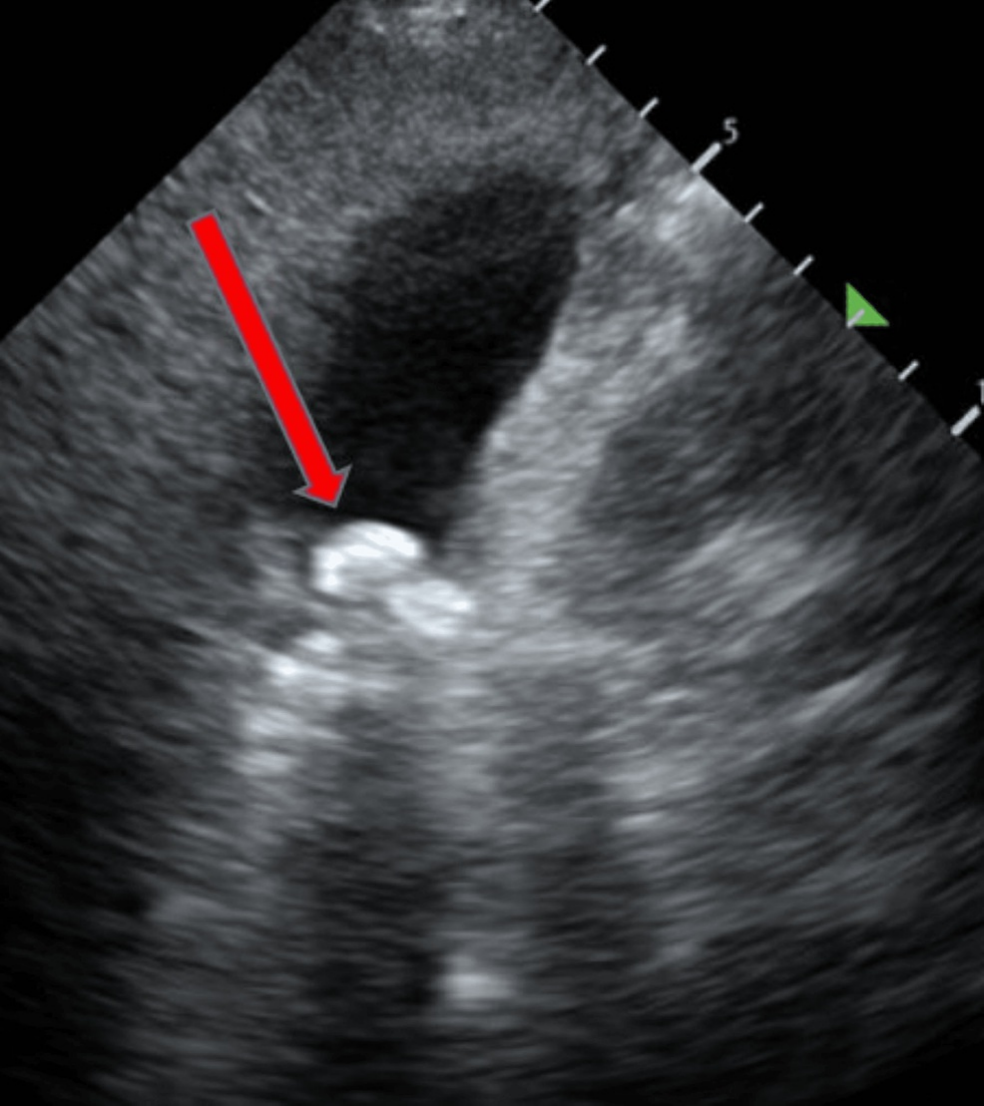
Ultrasound scan of the gallbladder showing multiple gallstones.
